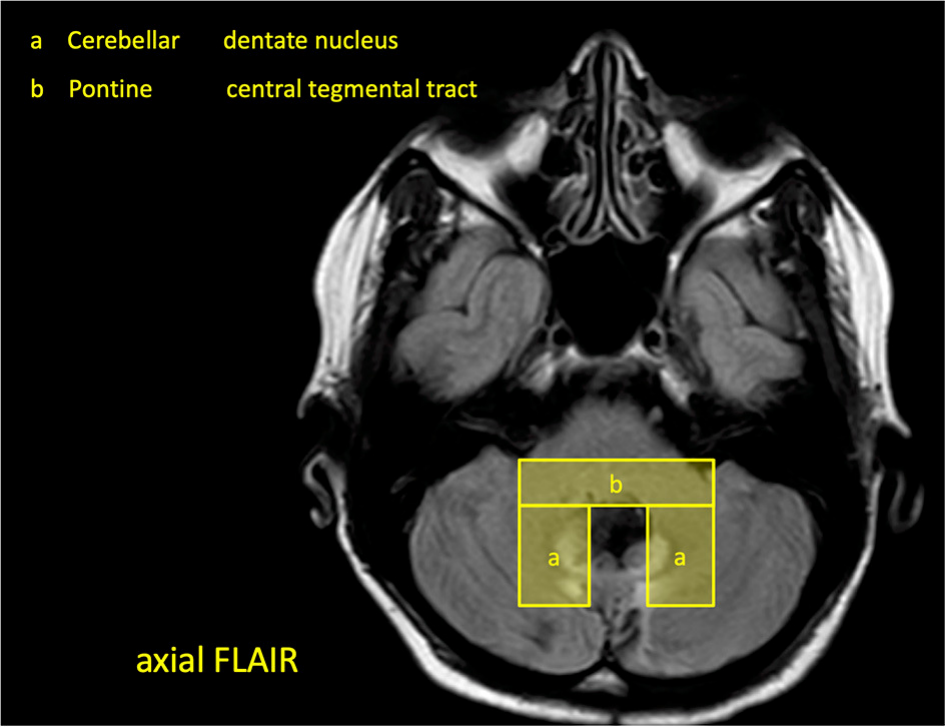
Excerpt of the study brochure, which shows an MRI template with the regions of interest.
